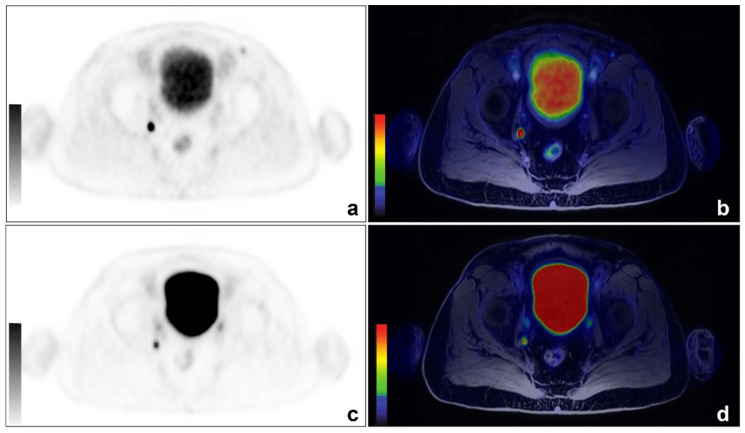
An example of concordant findings between ^68^Ga-PSMA and ^68^Ga-DOTA-RM2 PET/MRI in detecting PCa lymph nodal recurrence. A 66-year-old patient previously treated with radical prostatectomy for a Gleason 7 (4 + 3) PCa (pT3aN0) experienced biochemical recurrence (PSA: 0.53 ng/mL). ^68^Ga-PSMA PET/MRI images showed a focal right obturator lymph nodal uptake ((a): axial ^68^Ga-PSMA PET; (b): axial ^68^Ga-PSMA PET/MRI). The same finding is visible on ^68^Ga-DOTA-RM2 PET/MRI images (c): axial ^68^Ga-DOTA-RM2 PET; (d): axial ^68^Ga-DOTA-RM2 PET/MRI).
